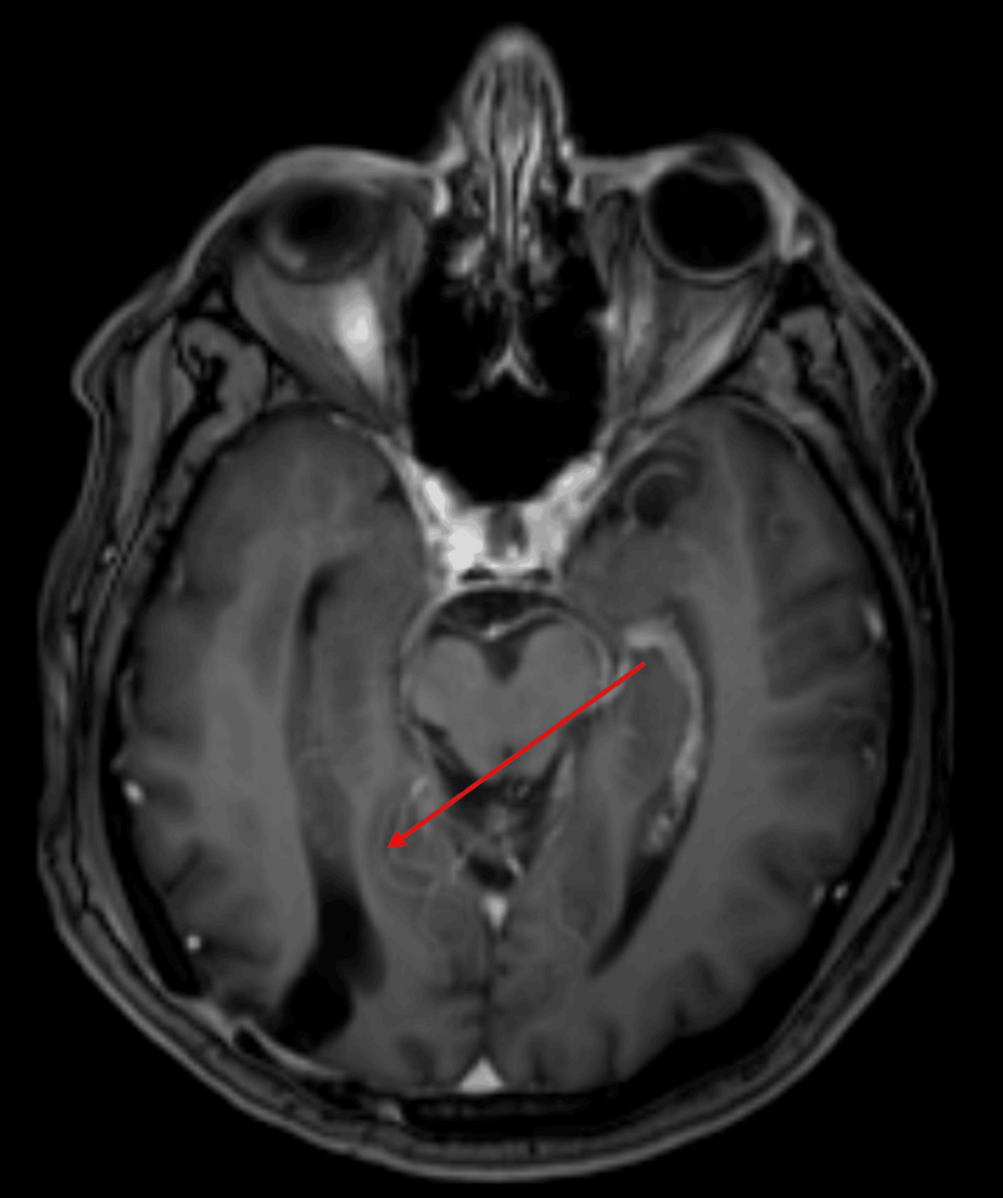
Axial MRI with areas of the right occipitoparietal gliosis without evidence of lesion recurrence.
